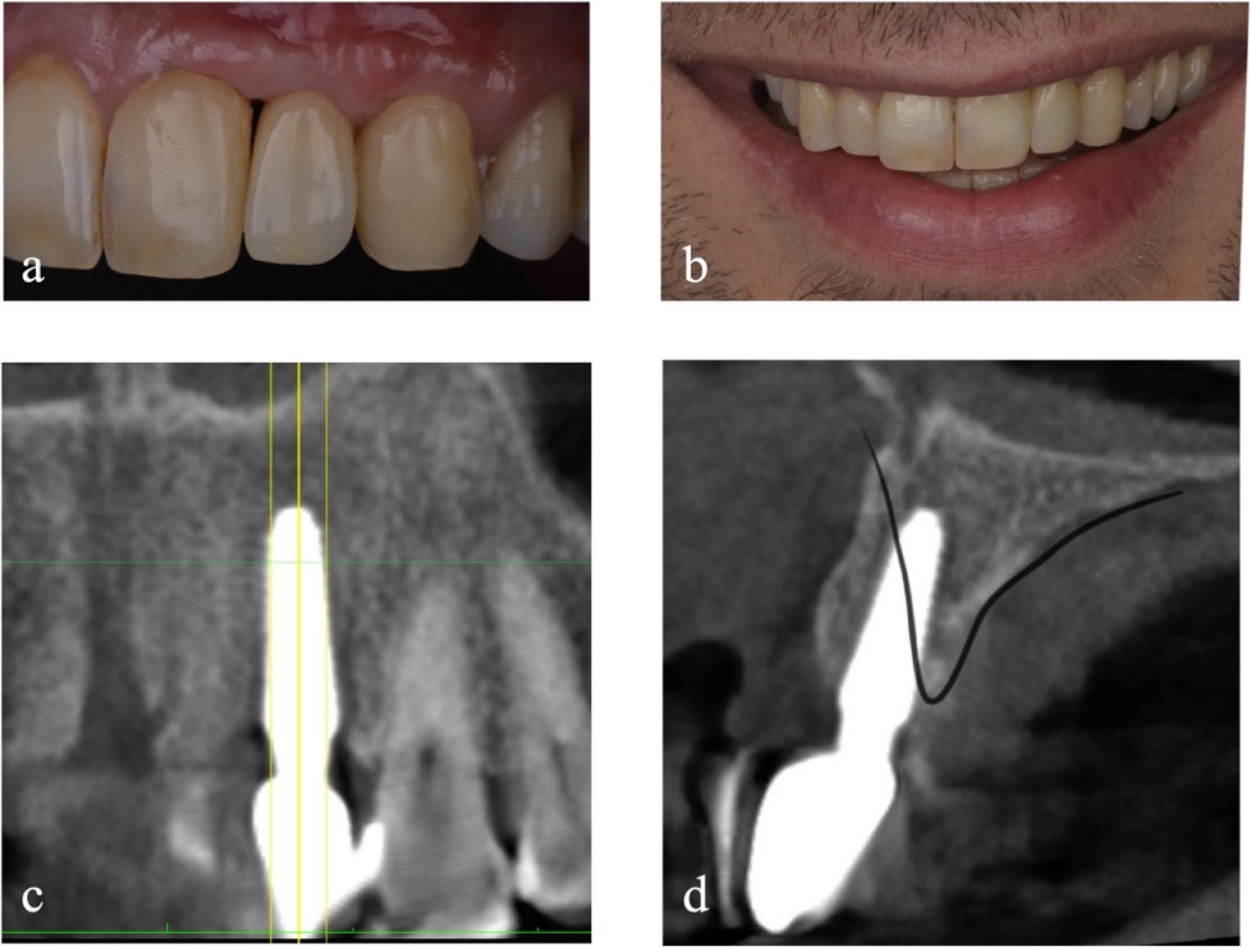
Intraoral (a) and extraoral (b) outcome of the rehabilitation at 3.5 years after the first surgery, with CBCT control (c, d)
